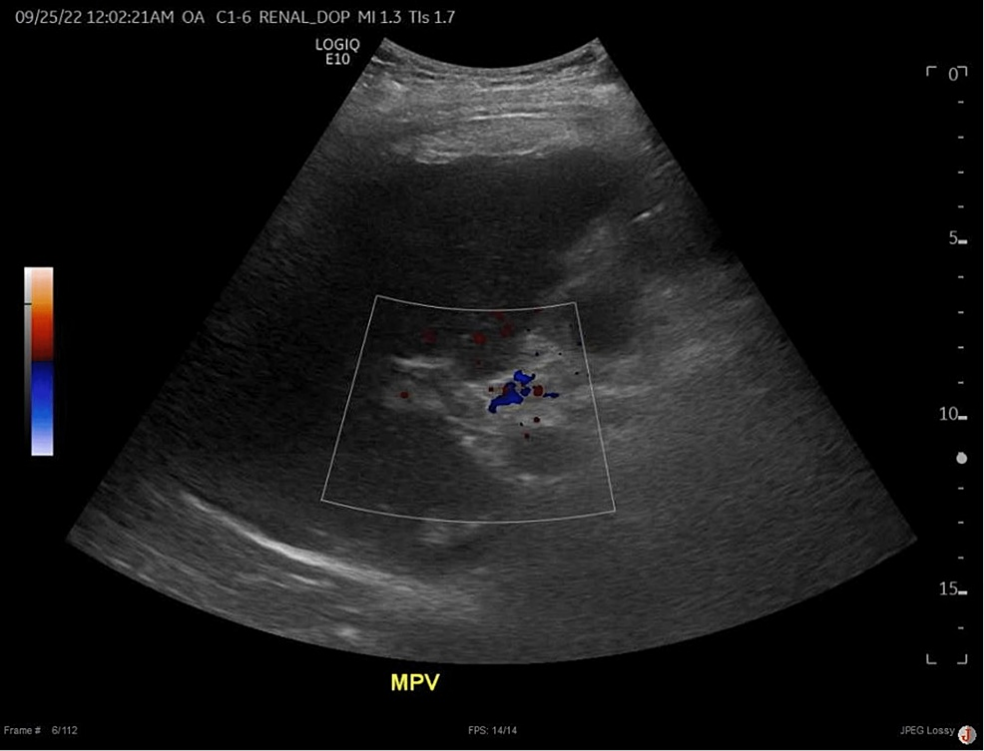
Repeat duplex ultrasonography eight days after the initial examination, revealing absent flow in the portal vasculature suspicious for a portal vein thrombosis. Hepatic vasculature remains patent with unchanged flow.
